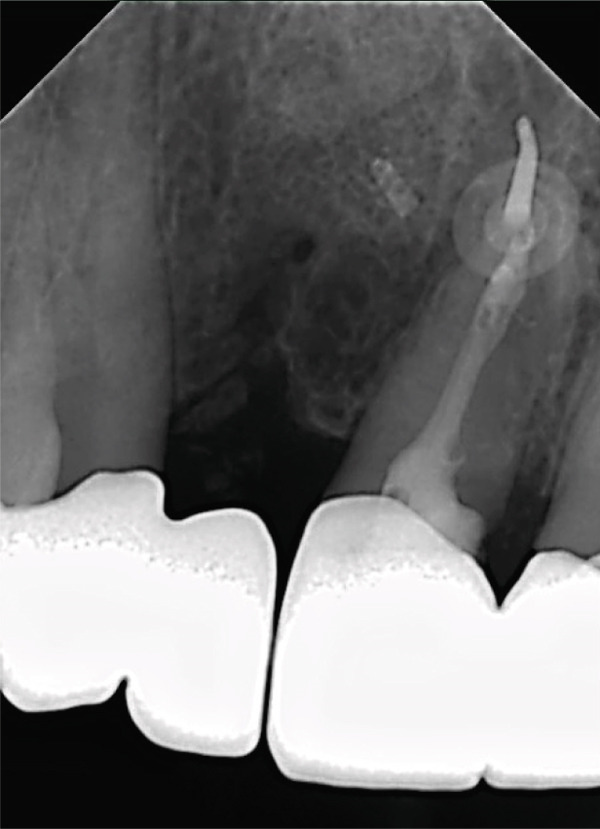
Radiographic evaluation demonstrating osseointegration and transplanted DAC stability.
